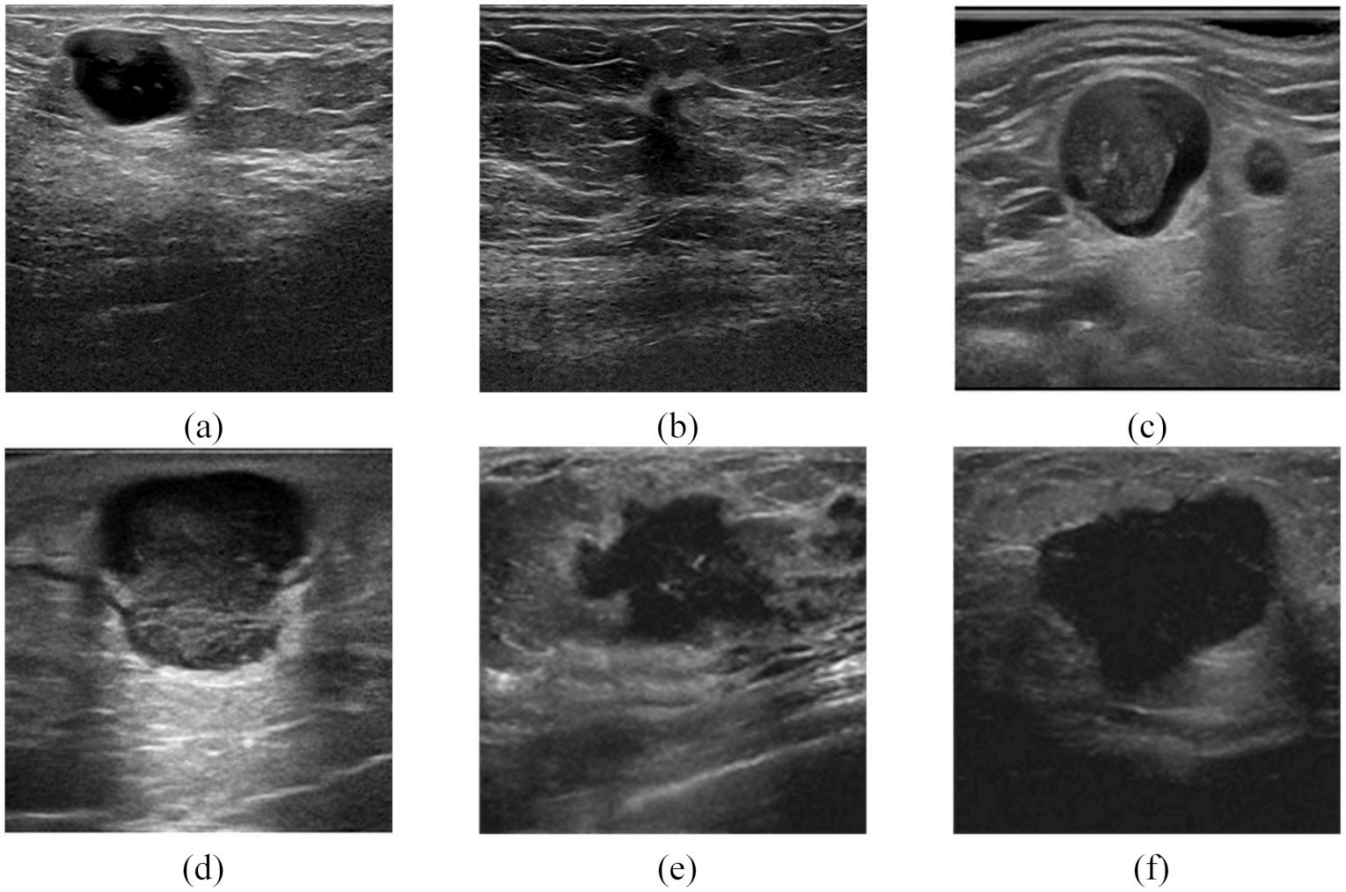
Benign and malignant tumors. (a) and (b) were obtained from Breast Ultrasound Lesions Dataset(Dataset B) [13]. (c) and (d) were acquired from Gelderse Vallei Hospital in Ede, the Netherlands [14]. (e) and (f) were obtained from the Imaging Department of the First Affiliated Hospital of Shantou University. It can be seen from these six figures that there are obvious differences between the tumor morphology and the surrounding tissues.
